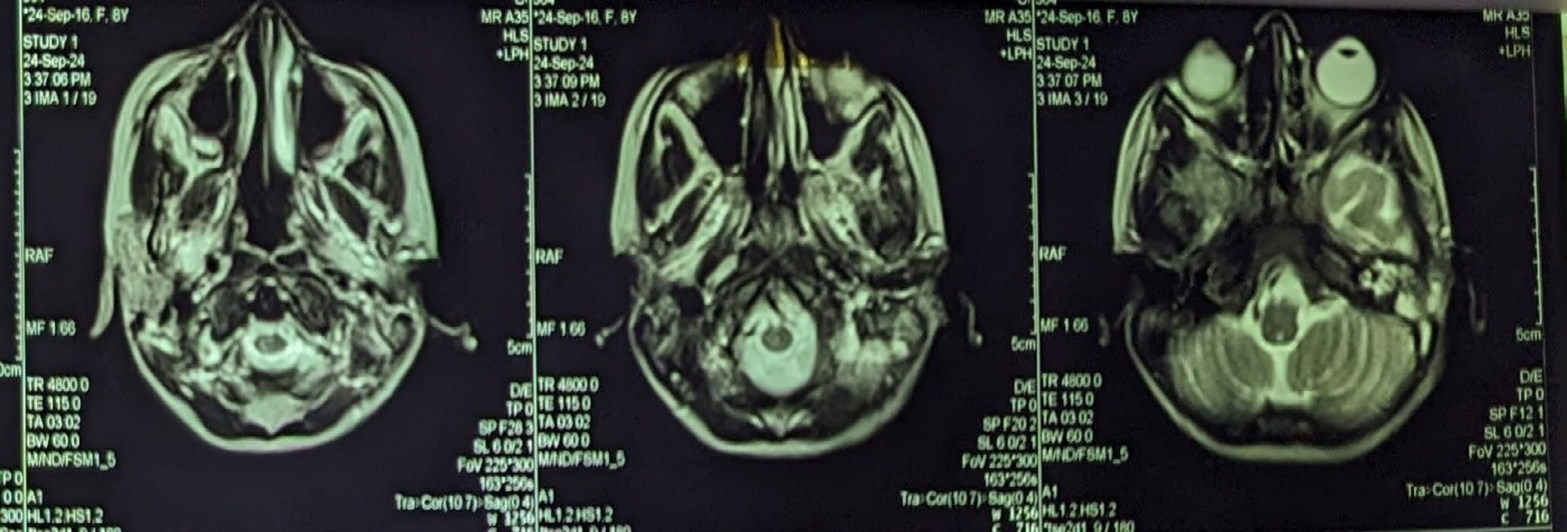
MRI showing loss of flow void in the left transverse and sigmoid sinuses, suggestive of thrombosis.
